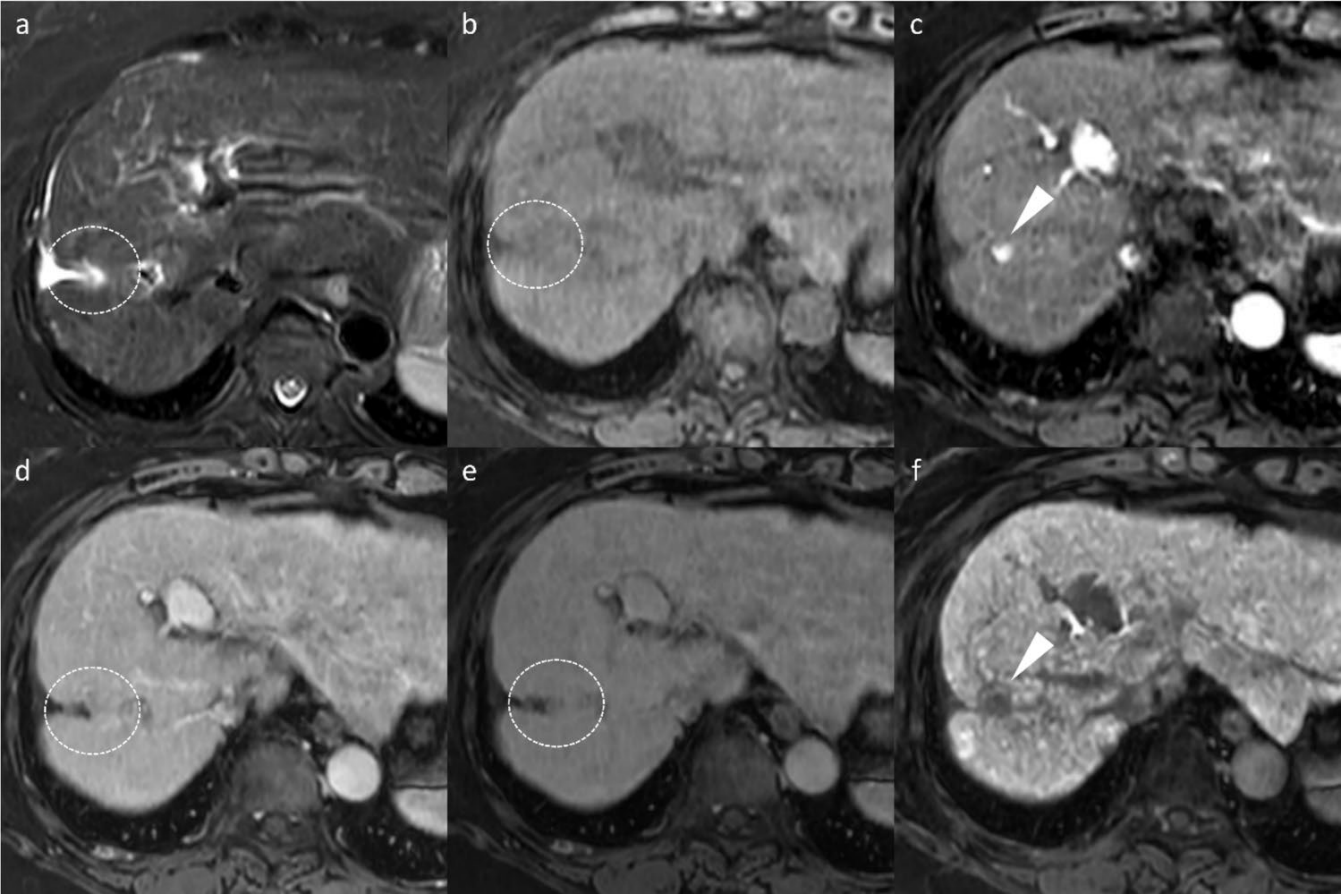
Recurrence of hepatocellular carcinoma after radiofrequency ablation (RFA) in a 75-year-old woman. A small focus of early arterial enhancement (arrowhead) adjacent to the post-RFA ablation zone raises suspicion for tumor recurrence (c). The lesion is inconspicuous on fat-suppressed T2-weighted imaging (a; dotted circle) and the pre-contrast image (b; dotted circle), and lacks definite portal venous washout (d; dotted circle) or hypointensity in the transitional phase (e; dotted circle). However, the clear hypointensity (arrowhead) observed in the hepatobiliary phase allows for a confident diagnosis of recurrence (f)
